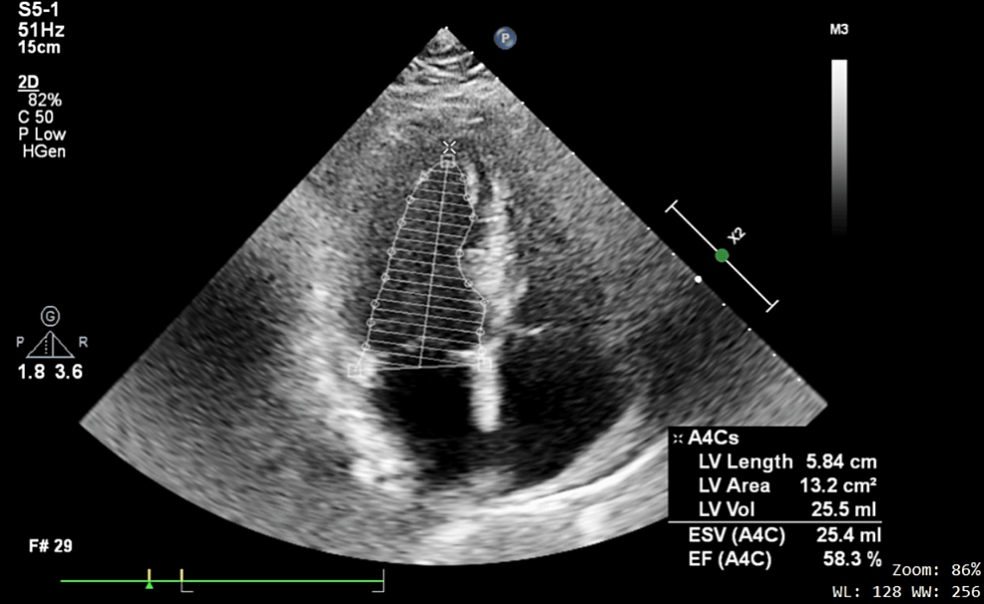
Transthoracic echocardiographic image showing normal left ventricular systolic function.
